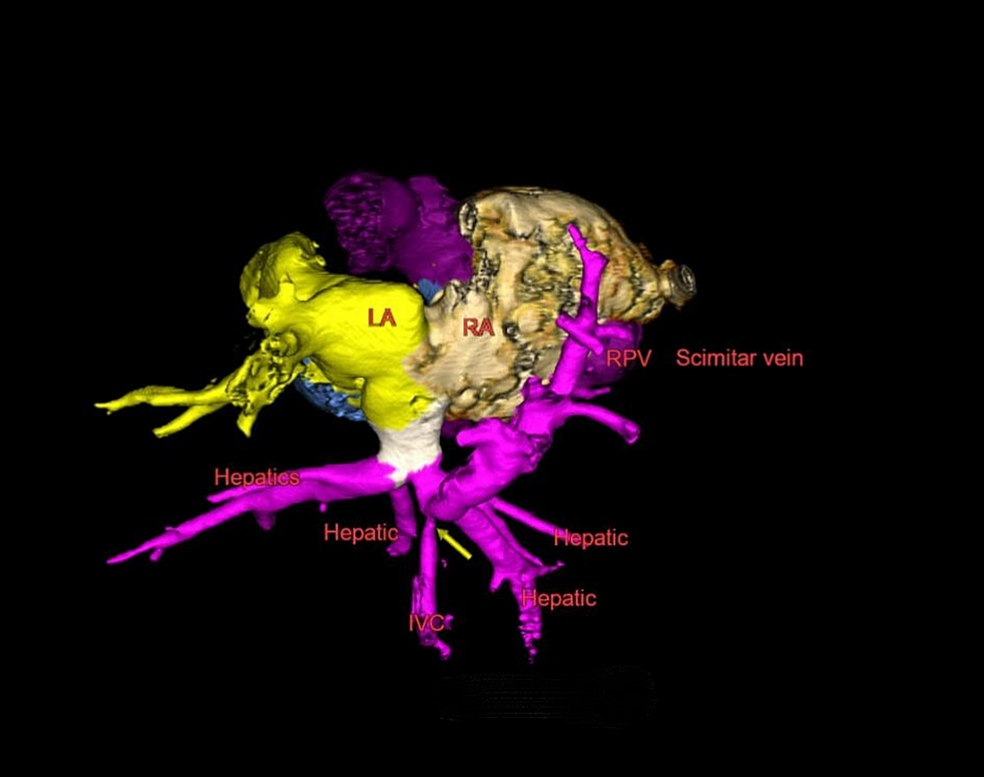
3D reconstruction of CTA images of scimitar vein and IVC demonstrating stenosis of scimitar vein at junction with right hepatic vein CTA = computed tomography angiogram, IVC = inferior vena cava, LA = left atrium, RA = right atrium, RPV = right pulmonary vein; Yellow arrow indicates area of stenosis.
